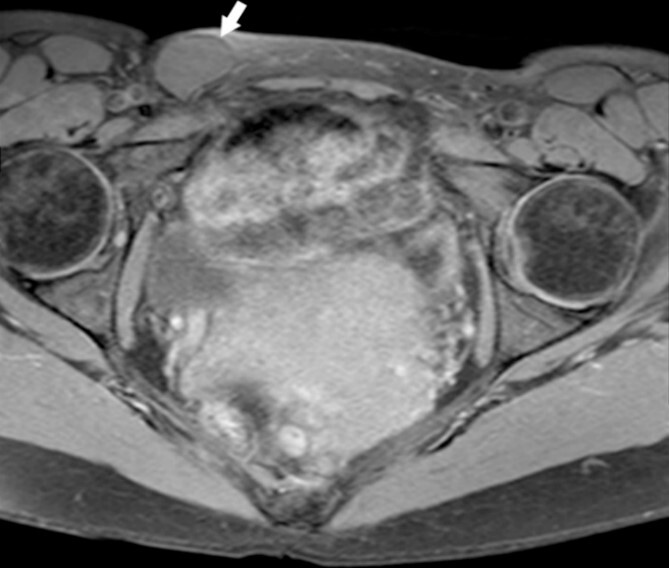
MRI of the Cyst of the Canal of Nuck–T2 sequence. This figure displays the cyst (indicated by the white arrow), showcasing its appearance on a T2-weighted image.
